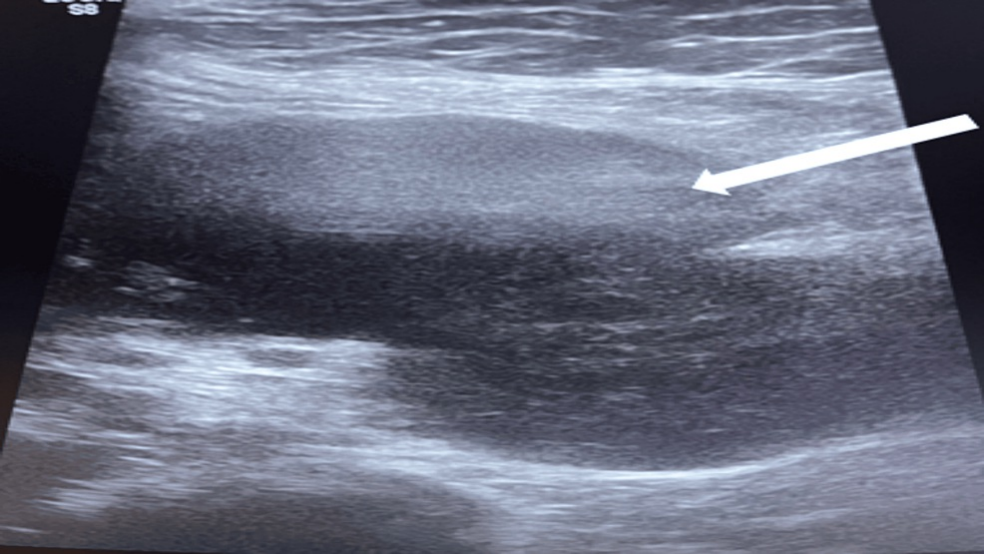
Ultrasound image showing a bilobed, hypoechoic, heterogeneous mesenteric mass (white arrow)
